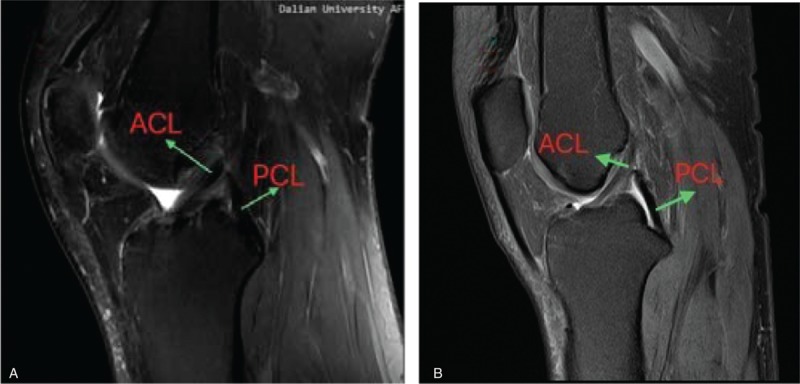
a: the ACL shape on MRI in patients postoperation; b: the ACL shape on MRI in healthy people. ACL = anterior cruciate ligament, MRI = magnetic resonance imaging.
